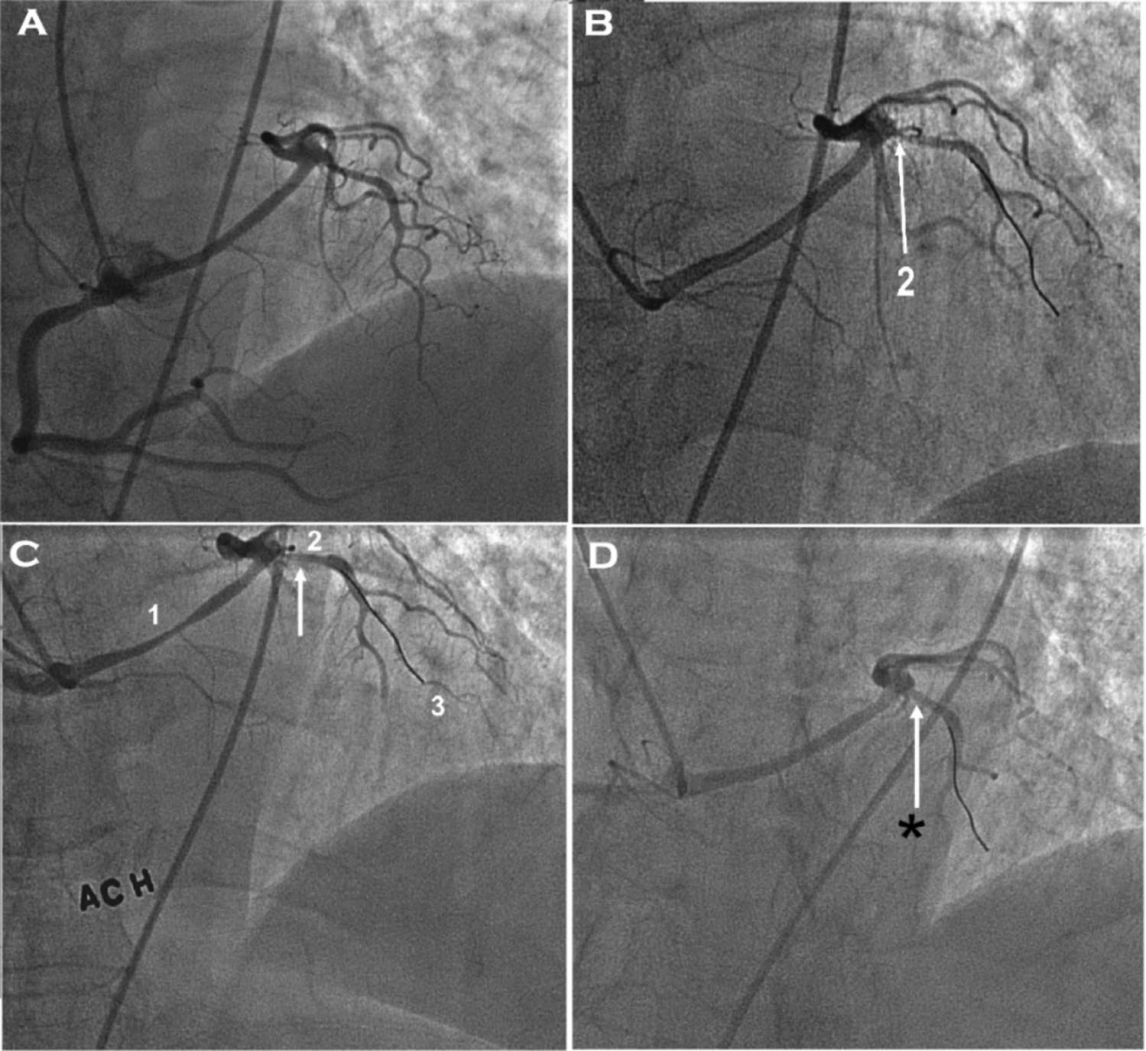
The influence of coronary guidewire advancement and acetylcholine administration in L-ACAOS-IS. A A patient with L-ACAOS-IS and spontaneous, recurrent angina at rest that required hospital admission is shown at baseline (previous computed tomography angiography and traditional coronary angiography; no wires). No luminal narrowing was seen in any segment. B Chest pain developed after a coronary pressure-wire was gently advanced to measure intracoronary gradient(s). The pressure sensor could not be advanced beyond the origin of the diagonal branch (spastic manifestations: site 2). A new angiogram showed localized de novo stenosis at a 90° curve (at the bifurcation of the diagonal branch, site 2). C After the coronary pressure-wire was pulled mildly, such that only the soft tip of the guidewire was at the spastic segment, the initial stenosis disappeared. A 100-mcg ACh test done 3 min later revealed new localized stenosis at the proximal left main segment (site 1) and distal left anterior descending segment (sites 2 and 3). D After nitroglycerin (100 mcg) was administered and the guidewire was pulled, all stenoses promptly disappeared. The unusual hyperactive coronary state was well documented at the onset of mechanical stimulation and ACh testing and responded promptly to removal of stimuli. Similar behaviors are frequently seen in L-ACAOS-IS patients who are predisposed to spontaneous angina at rest. *ACh* indicates acetylcholine, *L-ACAOS-IS* anomalous origin of the left coronary artery from the right sinus of Valsalva, with intraseptal course
